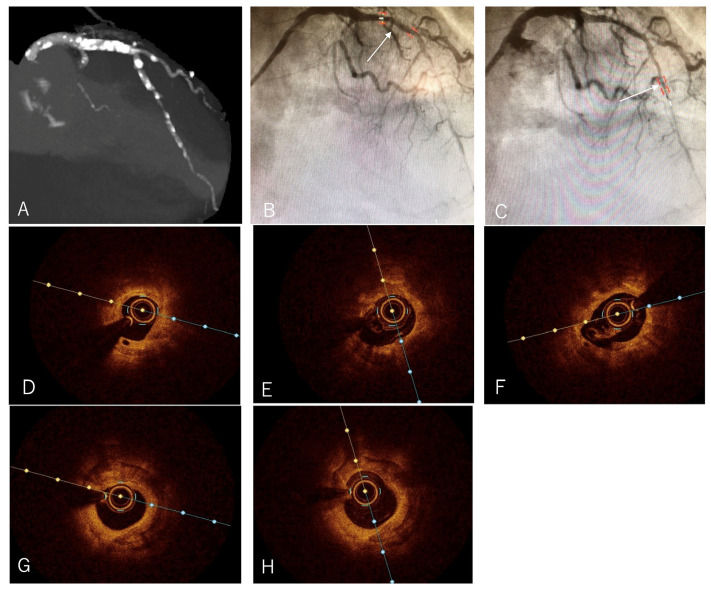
OA for a long lesion. (A) Computed tomography angiography (CTA). (B) OCT guidance identifying the proximal OA target site. (C) OCT guidance identifying the distal OA target site. (D) Proximal OCT before OA. (E) Proximal OCT after OA at 80,000 rpm. (F) Calcium thickness was still more than 800 μm at 10–11 o’clock so 120,000 rpm OA was applied to the proximal lesion. Proximal OCT after OA at 120,000 rpm. (G) Distal OCT before OA. (H) Distal OCT after OA at 80,000 rpm. Calcium thickness was less than 400 μm, so 120,000 rpm OA was not employed. White arrows indicate the lesion. Red lines indicate the modification area of OA.
